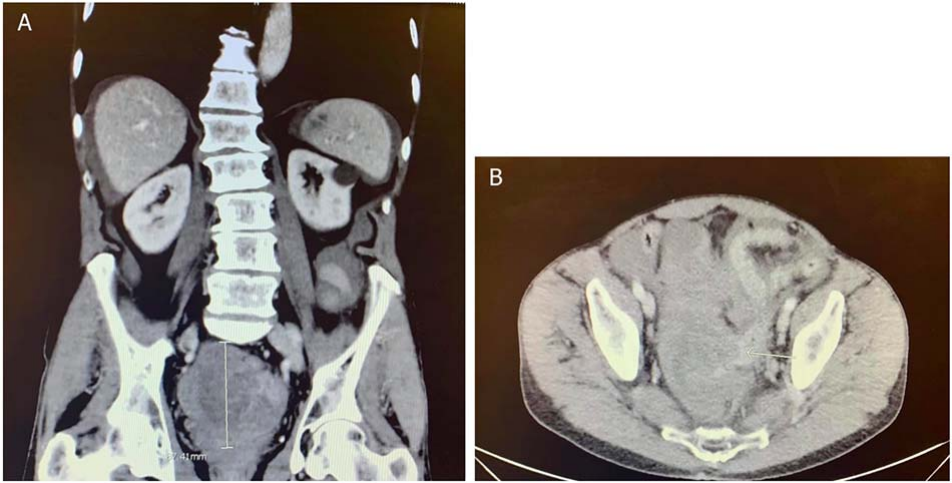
CT abdomen and pelvis with contrast in (A) anterior–posterior and (B) coronal views identified a rectovesical hematoma mass measuring 8.2 × 7 cm.
